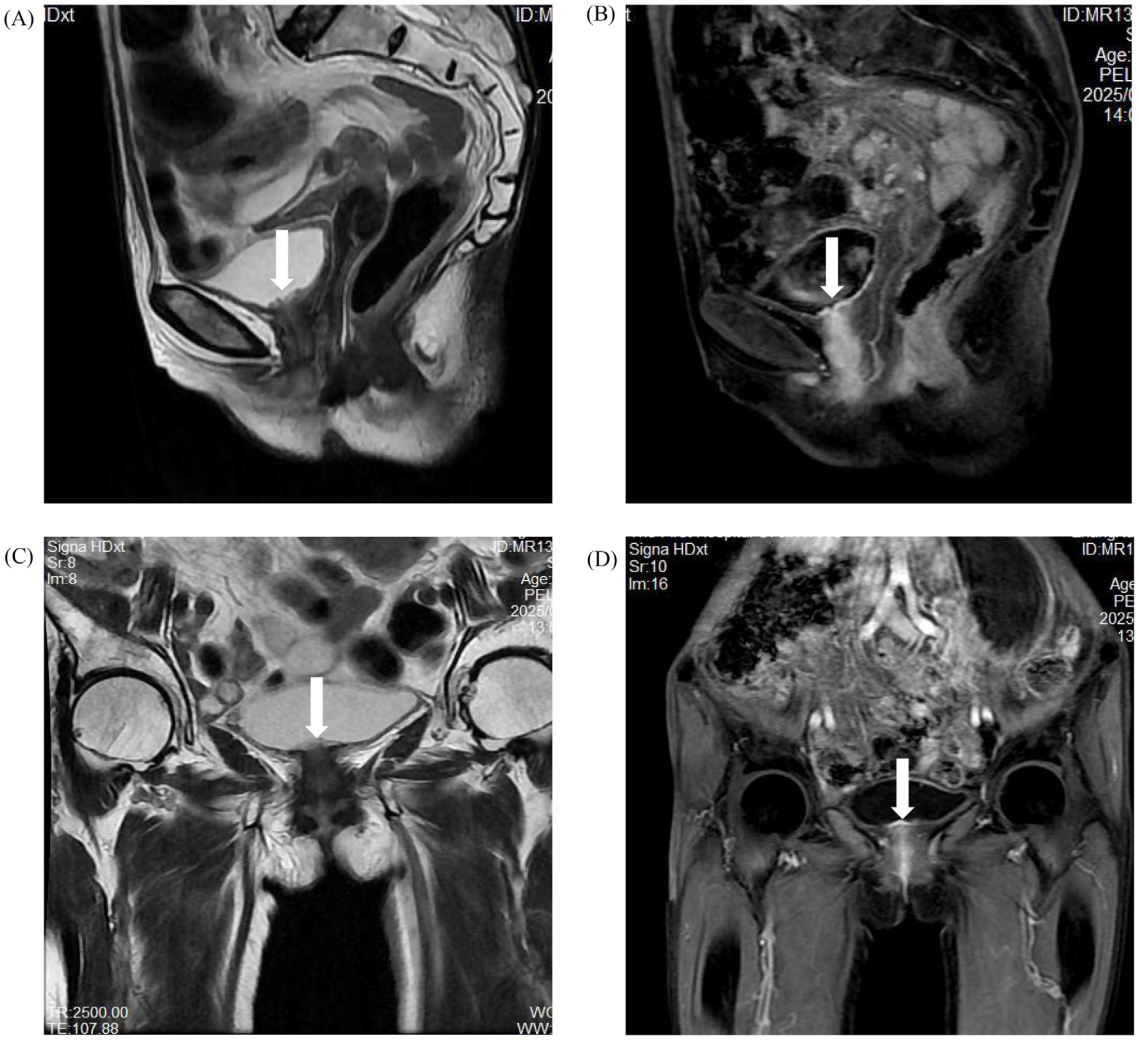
(A) Sagittal T2-weighted image (T2WI), (B) Sagittal contrast-enhanced T2WI (T2+C), (C) Coronal T2WI, and (D) Coronal T2+C demonstrate mild thickening of the bladder neck. A poorly defined multilocular cystic structure is observed inferiorly, resembling an enlarged male prostate in morphology, with slight upward protrusion into the bladder lumen. The lesion exhibits mild hyperintensity on T2WI, while contrast-enhanced imaging reveals prominent mucosal enhancement with persistent enhancement pattern and mild muscular layer enhancement. The lesion is indicated by the white arrow.
